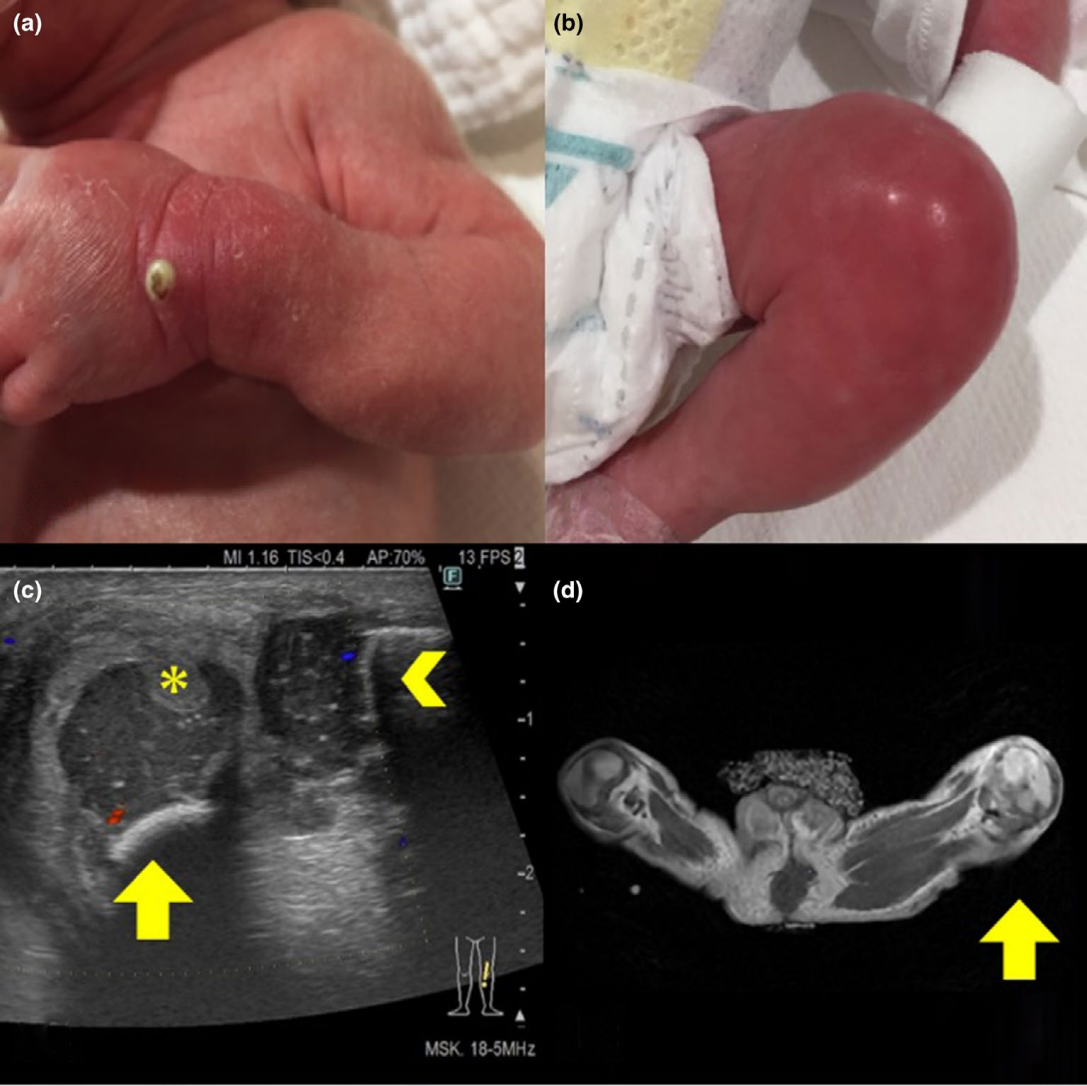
(a) On the day of life 23, redness and swelling accompanied by an accumulation of pus were found on the infant's left hand where peripheral venous catheter had been placed from the day of life 18 to 21. (b) On the day of life 28, redness and swelling appeared on the neonate's left knee. (c) Ultrasound examination performed on the day of life 43 shows femoral epiphyseal cartilage (arrow) and tibial epiphyseal cartilage (>). Increased blood flow signal is seen around the knee joint and within the epiphyseal cartilage compared to the contralateral side. Thickened synovial membrane in the anterior region partially erodes into the femoral epiphyseal cartilage (*). Inflammation from the arthritis of knee joint is extended to the distal epiphyseal cartilage implying chondritis. (d) Magnetic resonance imaging performed on the day of life 41 shows the extension of inflammation to the cartilage on the neonates's left knee (arrow) compared to the right.
